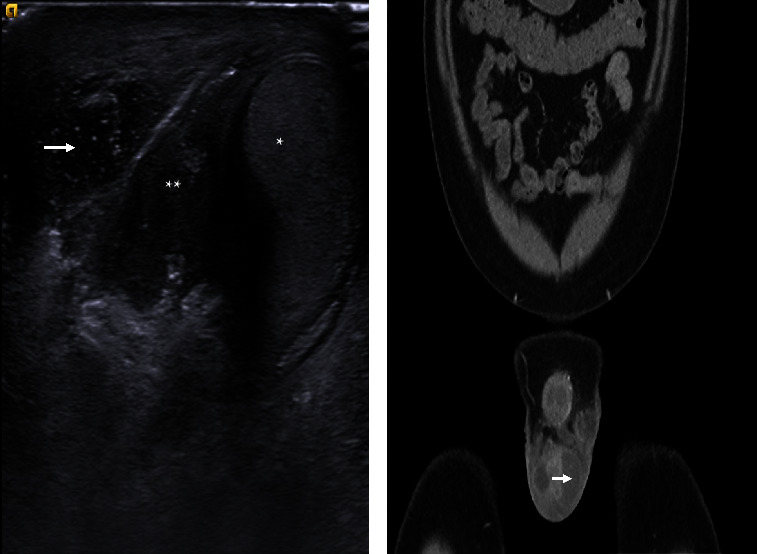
Diagnostic imaging. (a) Scrotal ultrasound depicting hypoechoic 5.5 cm × 3.6 fluid collection (i.e., abscess; arrow) adjacent to epididymis (double star) and testis (star) in the right hemiscrotum. (b) Coronal CT abdomen/pelvis with contrast depicting scrotal abscess formation as suggested by presence of rim-enhancing fluid collection (arrow).
